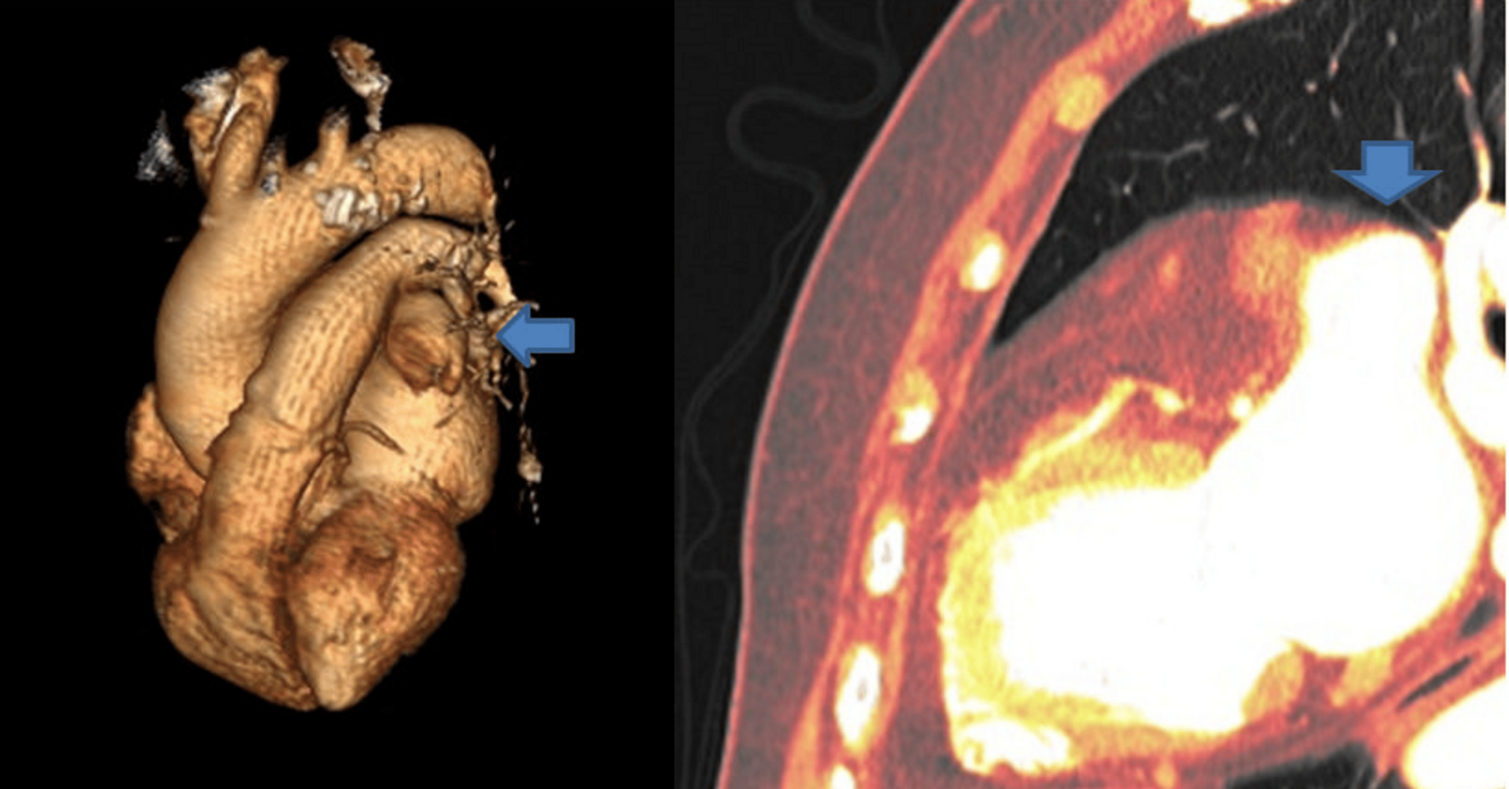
Cardiac CT 3D reconstruction image; left atrial appendage aneurysm (blue arrow)
